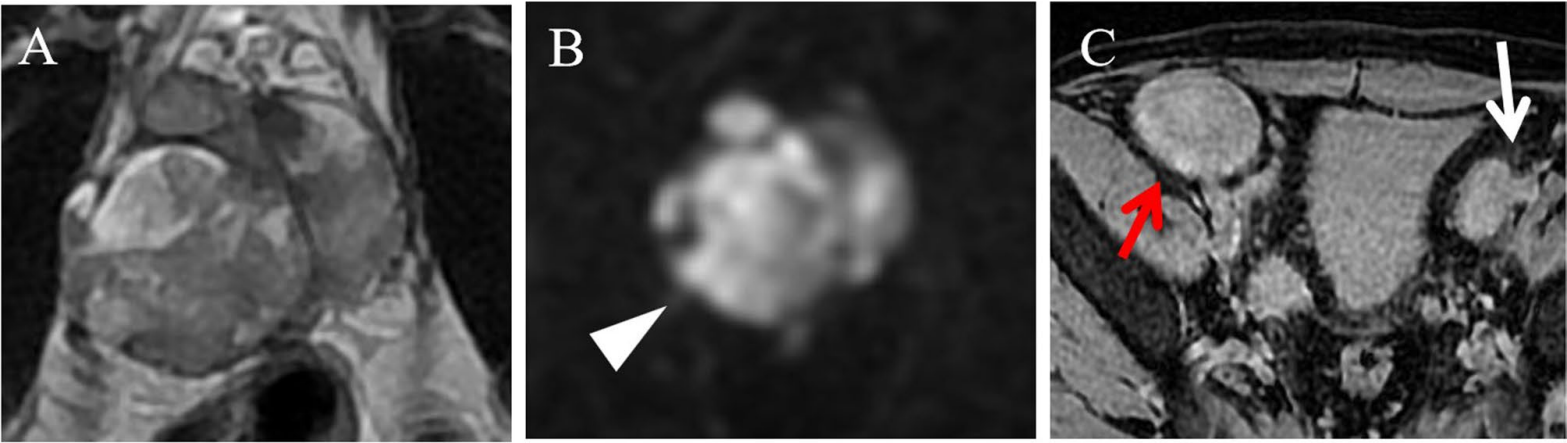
MP-MRI indicates an enlargement of the lesion scope compared to the previous scan (A), with pronounced diffusion restriction (white triangle, B). Enlarged lymph nodes are observed adjacent to the left iliac vessels (white arrow, C). The red arrow points to the transplanted kidney
